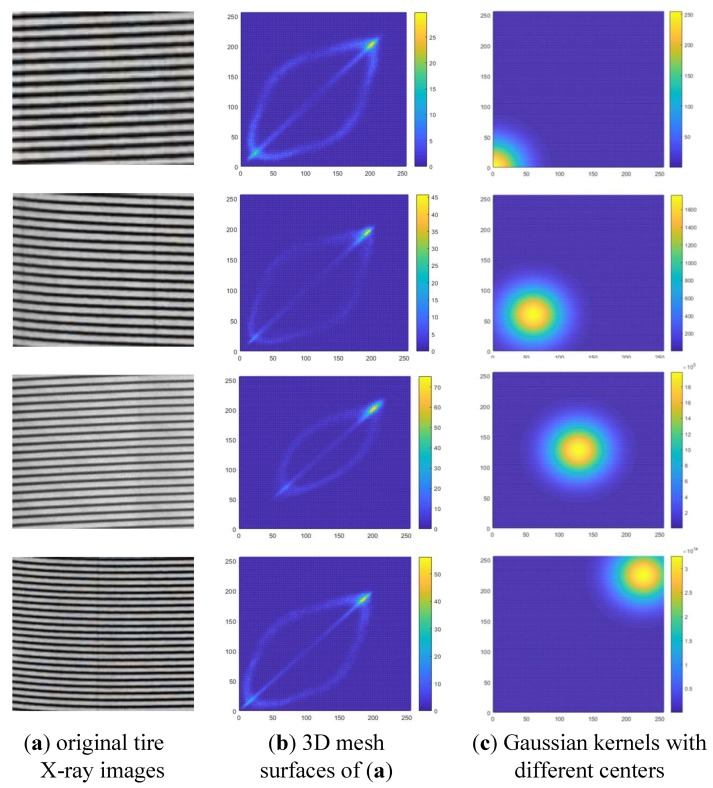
Exhibition of characteristic analysis of different tire X-ray images.
